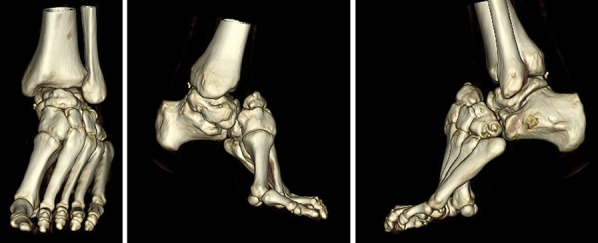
Three-dimensional CT representations of the left foot and ankle demonstrating notable equinovarus deformity with chronic dislocation of the Chopart joint and resultant talus and navicular dysplasia.
